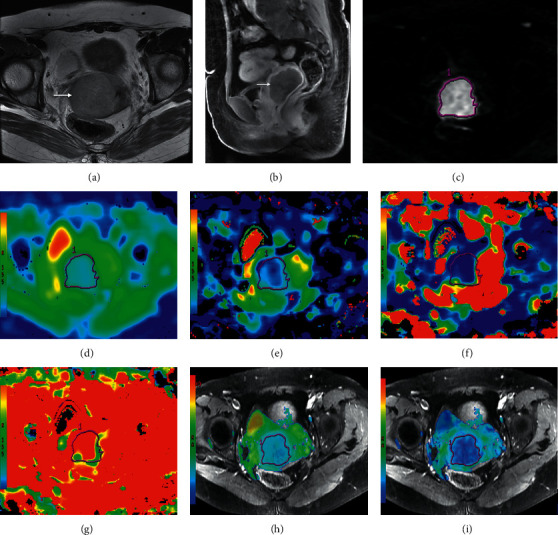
A 52-year-old woman with stage IIA cervical cancer. (a) is T2-weighted image (T2WI) shows a slightly hyperintense cervical mass, which was slightly enhanced on the contrast-enhanced magnetic resonance image (b). (c) shows the tumor with hypertension on the IVIM-DWI image with the *b* value of 800 s/mm^2^. (d–g) display the ADC, D, D ^*∗*^, and *f* parametric maps are derived from IVIM-DWI. (h–i) show the DCavg and FA maps derived from DTI.
